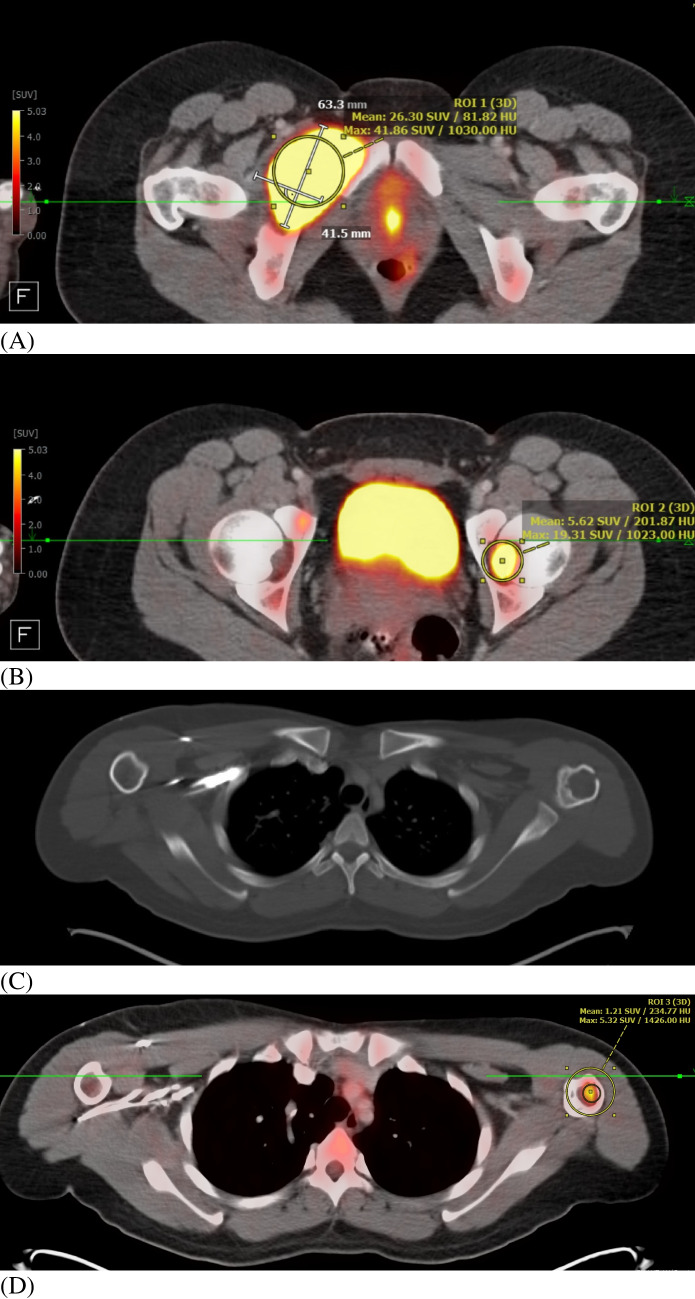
FDG PETCT with diagnostic CT. A Lytic and soft tissue lesion involving the right superior pubic ramus and anterior acetabulum with a large soft tissue component extending into the adductor muscles and extending into the anterior inferior aspect of the hip joint/acetabular fossa with intense FDG uptake, measuring up to 63 × 42 mm SUVmax of 41.9. B Soft tissue lesion centered in the left femoral head with cortical breakthrough and involvement of the left hip joint with intense FDG uptake SUVmax of 19.3. C Lucent lesion with focal intense FDG uptake and focal cortical breakthrough in the left proximal humerus (arrow). D Left proximal humerus lesion demonstrates focal intense FDG uptake SUVmax of 5.3
